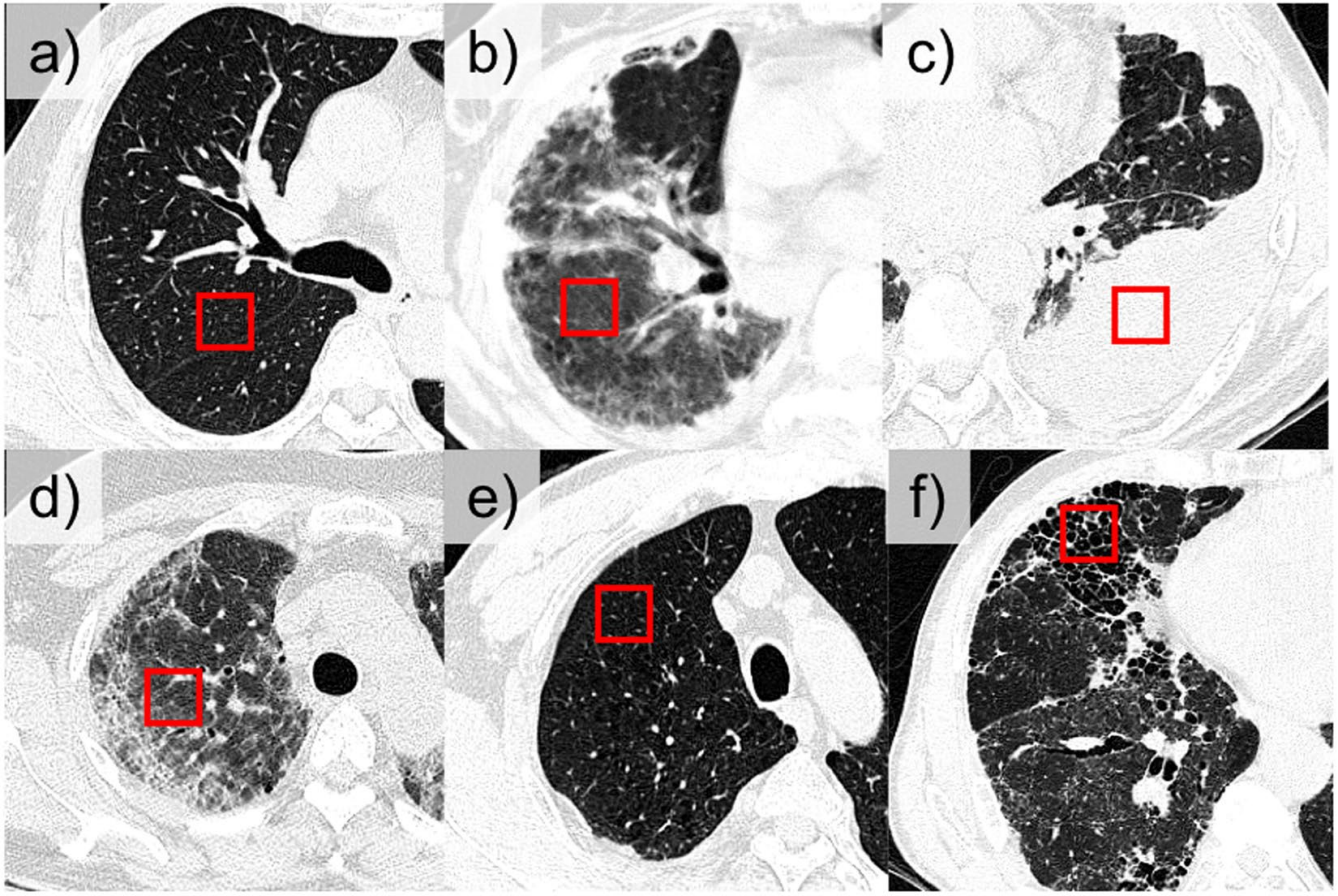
Examples of 2D HRCT lung parenchyma images with ROIs (red box) demonstrating (a) normal, (b) ground-glass opacity, (c) consolidation, (d) reticular opacity, (e) emphysema, and (f) honeycombing patterns.
